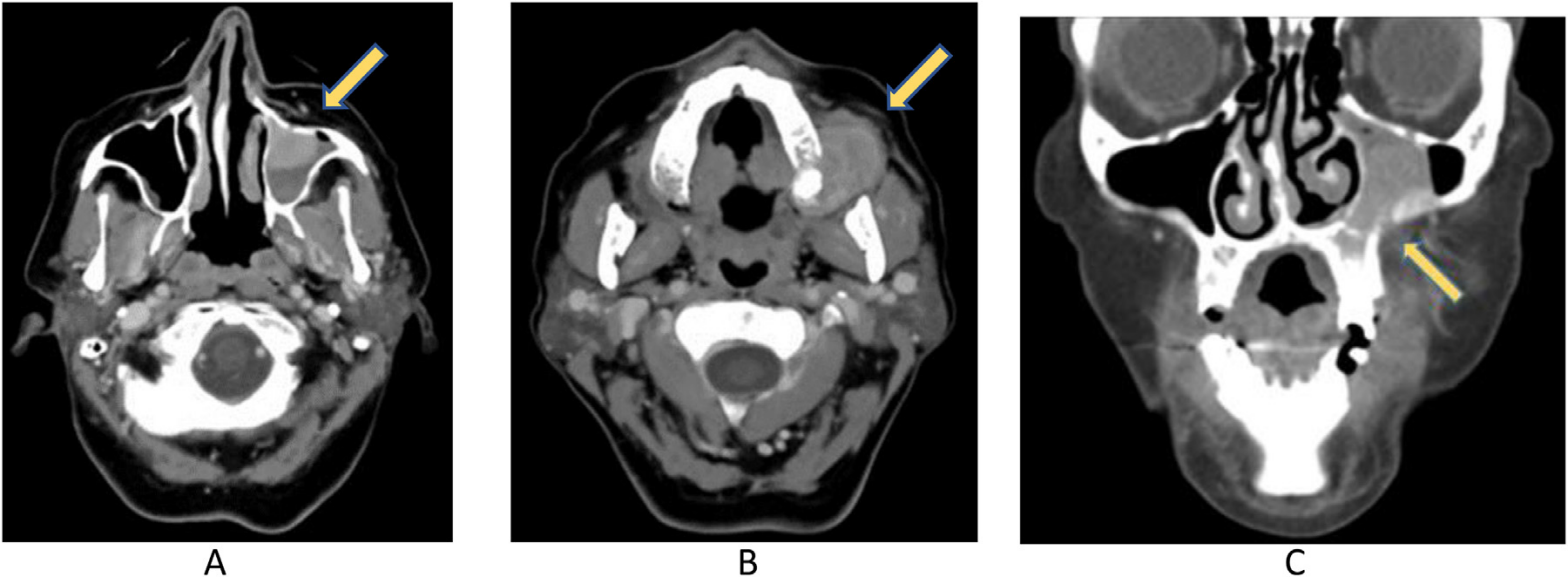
(A-C): enhanced facial CT scan axial plane (A,B) revealed the presence of a soft tissue density lesion in the left maxillary sinus. The lesion showed inhomogeneous contrast-enhancement after iodinate contrast agent administration. Coronal plane (C) image suggested the presence of erosion of the left maxillary sinus floor and extension of the mass to the mouth.
